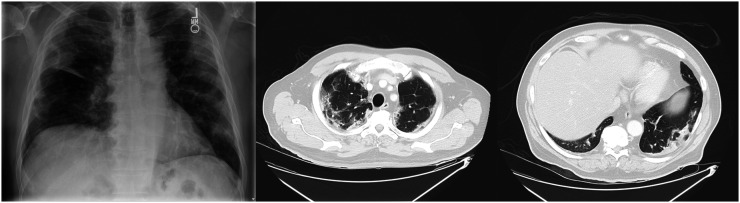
Initial chest radiograph at hospital admission showing diffuse pulmonary opacities in mid and peripheral lungs bilaterally (left), and transaxial CT scans of upper lung (middle) and lower lung (right) showing areas of ground-glass and consolidative changes on day 4 after admission.
